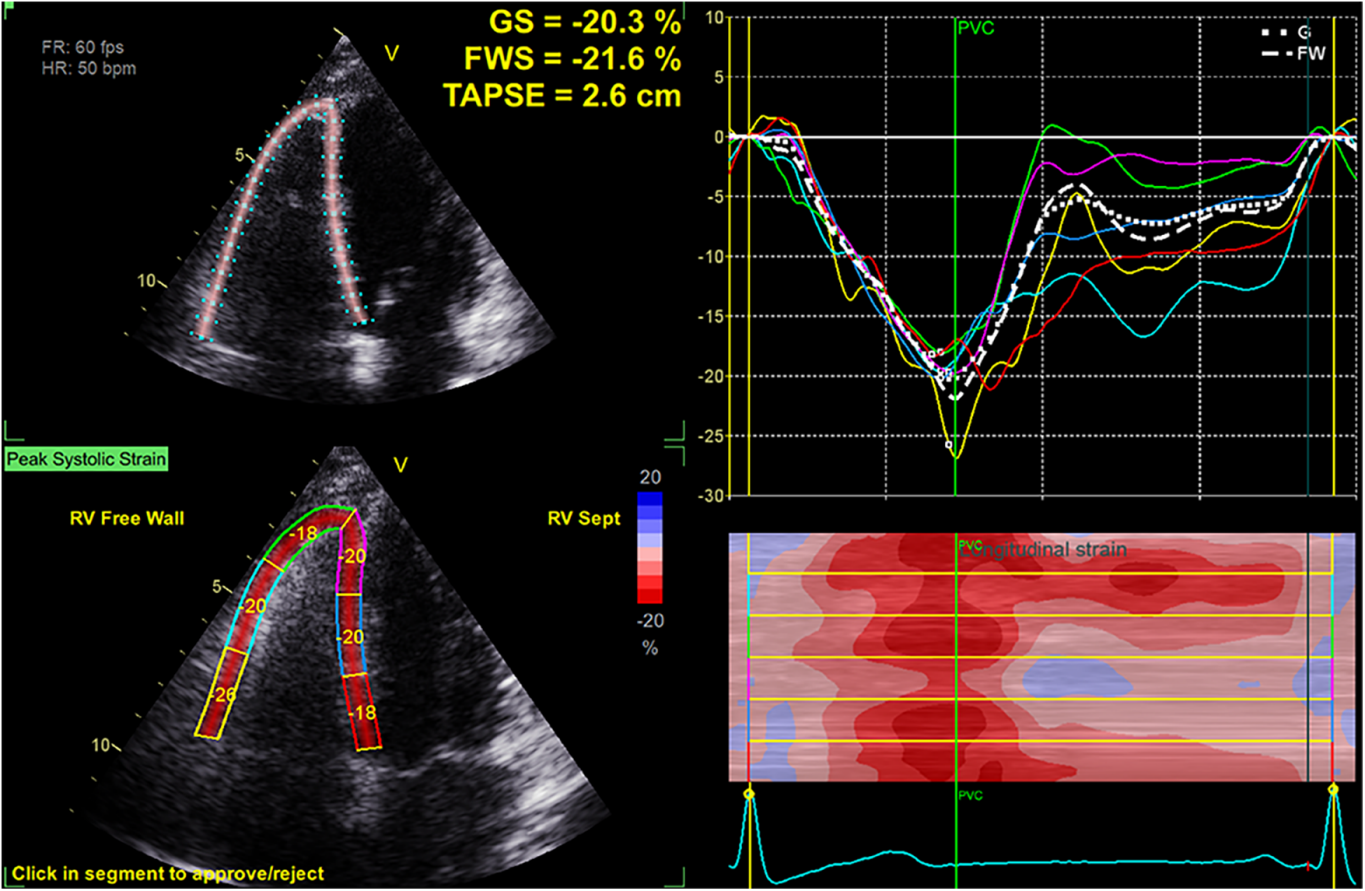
Measurement of RV-FWS and RV-GS in an RV-focused apical four-chamber view.
